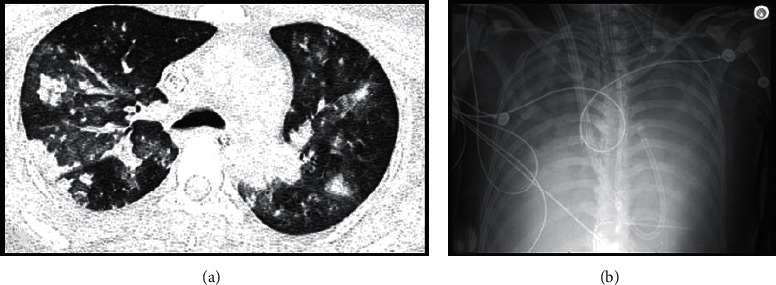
(a) High-resolution computed tomography with centrilobular and peripheral consolidation and surrounding ground-glass opacities compatible with pulmonary hemorrhage. Small basilar dependent pleural effusions were also present (not pictured). (b) Chest radiography showing near complete opacification of the lungs in the setting of diffuse alveolar hemorrhage.
